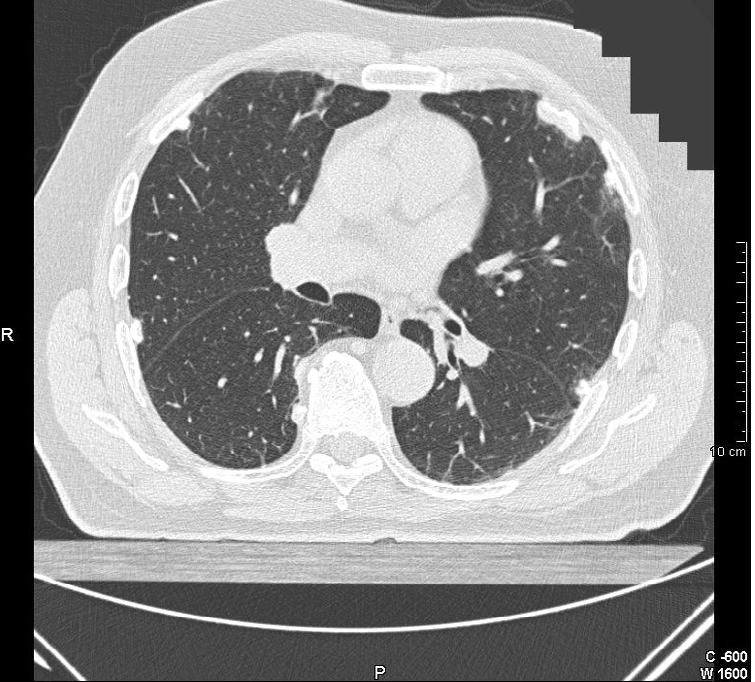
CT scan of the thorax demonstrating circumscribed calcified bilateral pleural plaques.
